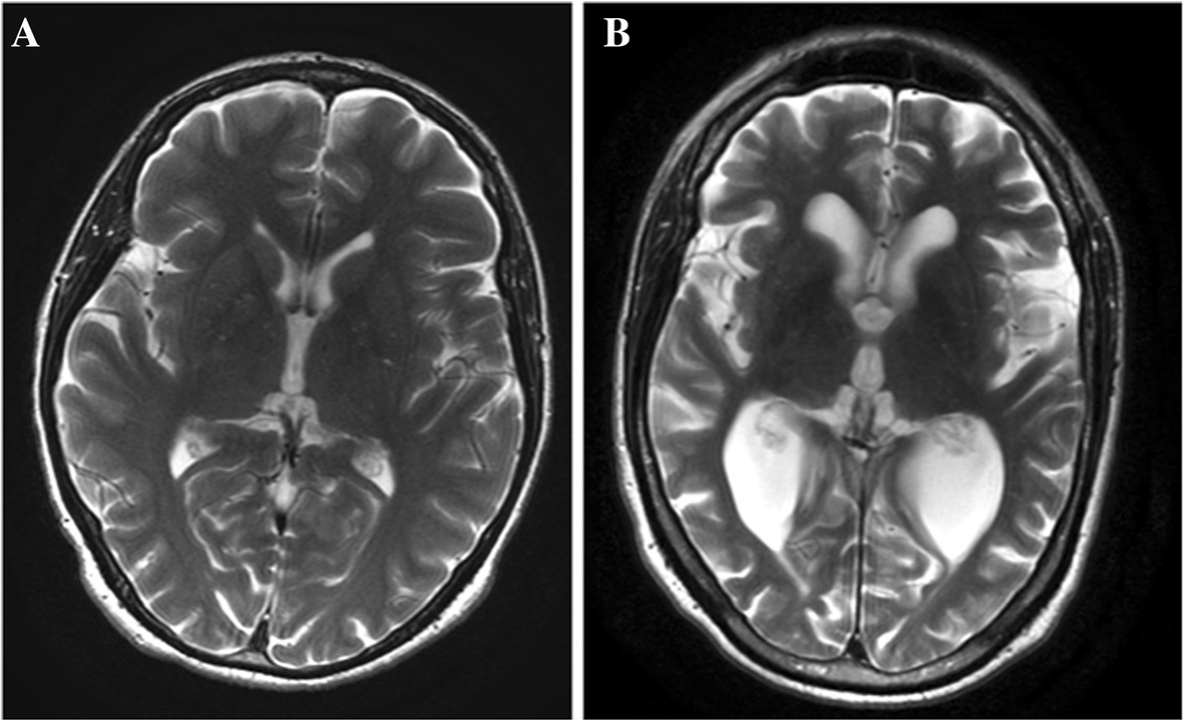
Brain MRI. Axial T2 images shows cerebral atrophy in patient with Alzheimer’s disease (A) and normal brain in control patient of same age (B).
